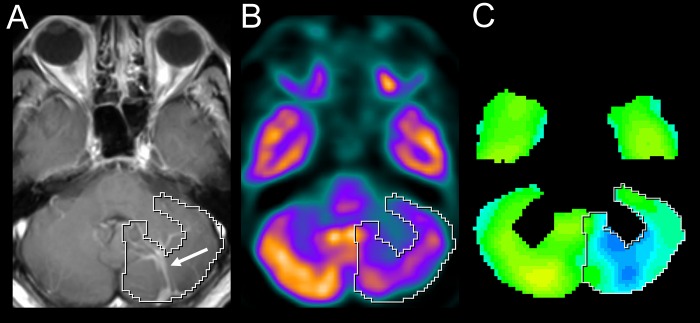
Representative axial MR (A), FDG-PET (B), and statistical map (C) of DVA-affected brain. A) Post-contrast T1 image shows a DVA (white arrow) within the left cerebellar hemisphere. B) FDG-PET demonstrates the DVAAh, qualitatively classified as severe, that corresponds to an SUVR of 0.65. C) The statistical map graphically depicts the standard deviations of the metabolic activity relative to a normal subject database. In this case, the auto-segmented region (white outline) of the left cerebellum corresponds to -3.6 standard deviations from the mean SUV in this region for normal controls.
